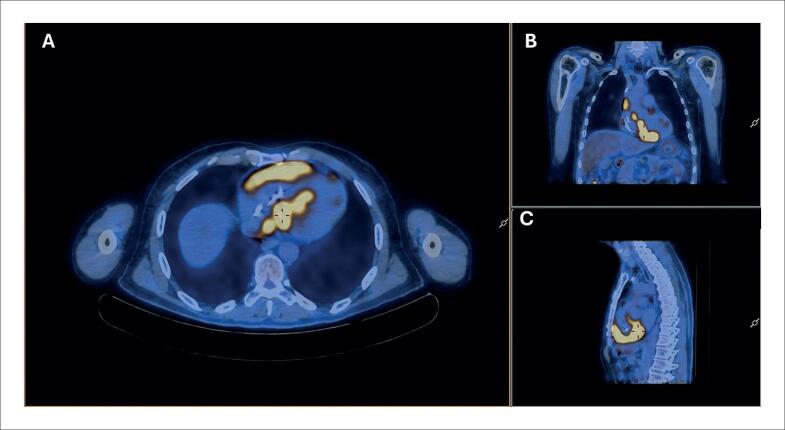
^18^F-FDG PET/CT em paciente com suspeita de sarcoidose cardíaca. (A) Corte axial demonstrando captação focal e heterogênea de ^18^F-FDG no miocárdio do ventrículo esquerdo, predominante no septo interventricular, com extensão para a parede do ventrículo direito, compatível com inflamação ativa. (B) Reconstrução coronal evidenciando a extensão da captação miocárdica. (C) Reconstrução sagital confirmando o padrão focal de hipermetabolismo miocárdico.
